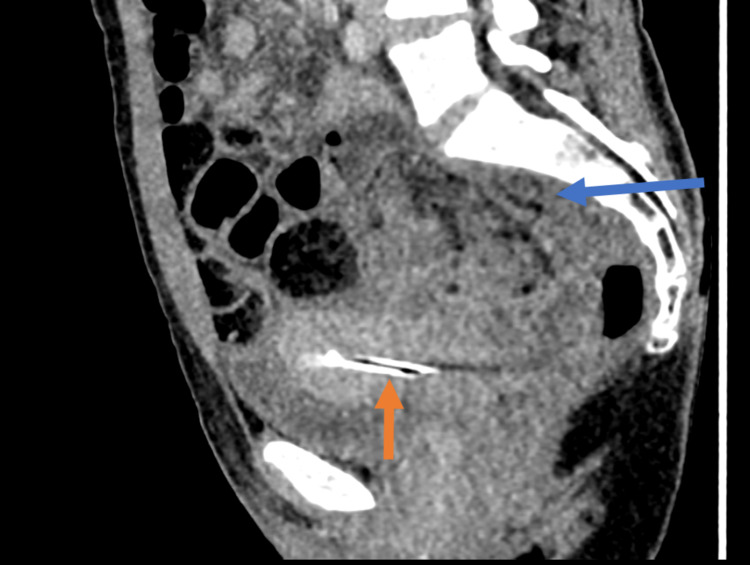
Sagittal portal venous phase CT of the abdomen and pelvis. Note the IUD in situ (orange arrow) and the presacral fat stranding (blue arrow). IUD: intrauterine device.
